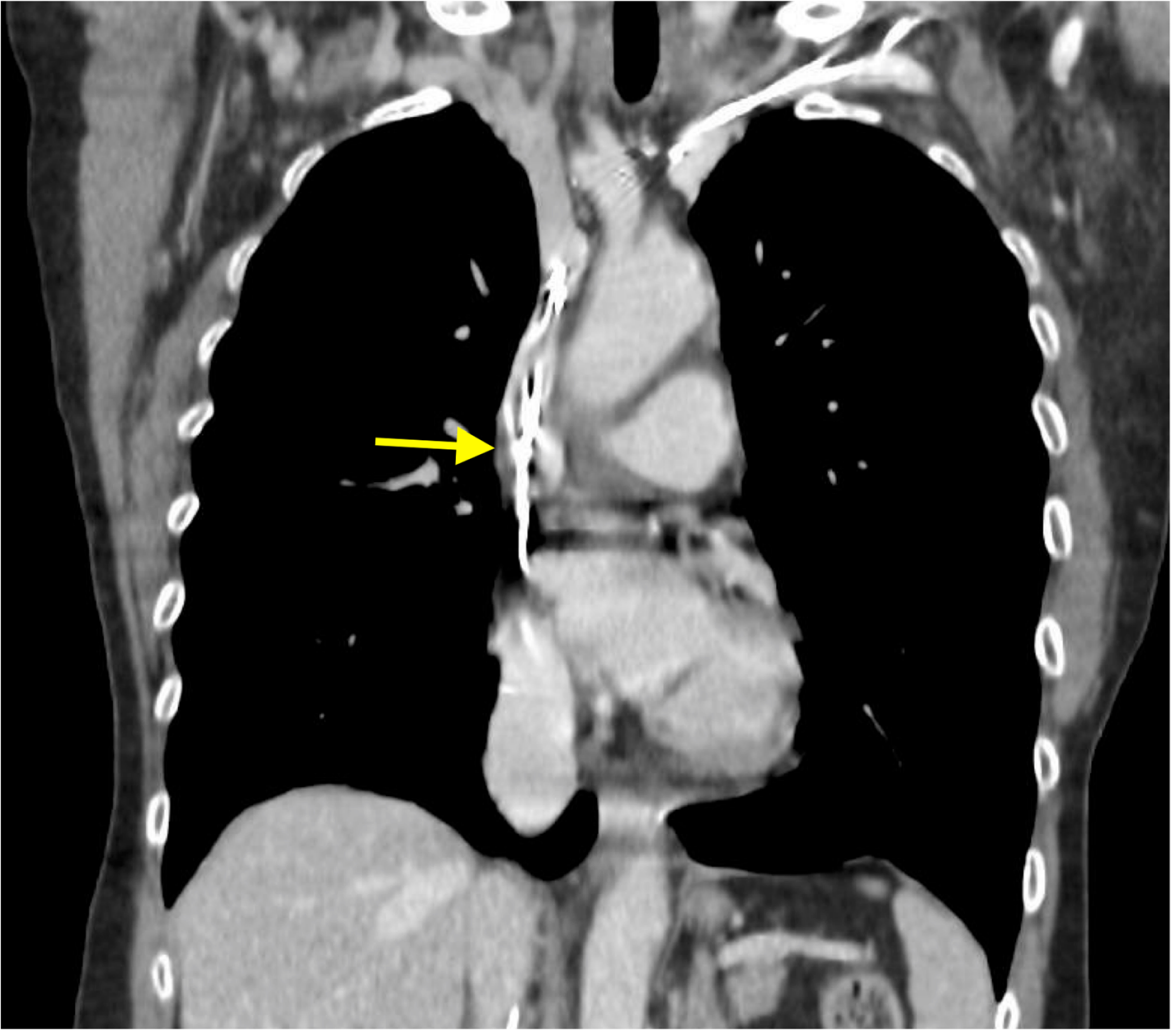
Thoracic CT angiography in coronal view, showing a superior vena cava obstruction determined by thrombus (arrow) around indwelling leads
